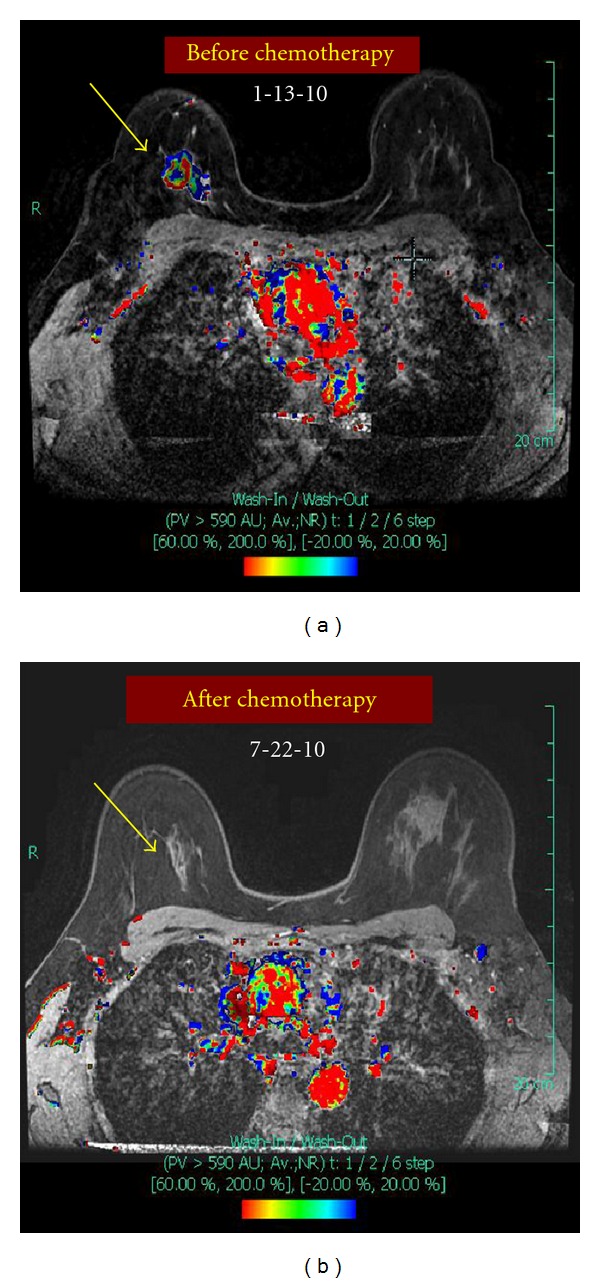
Comparison of bilateral breast MRI studies performed before and after neoadjuvant chemotherapy. (a) Doppler flow MRI before neoadjuvant chemotherapy. Yellow arrow points to the 2.7 cm irregular mass in the right breast. (b) Doppler flow MRI after neoadjuvant chemotherapy shows complete radiologic response. Yellow arrow points to area where the mass was located prior to treatment.
